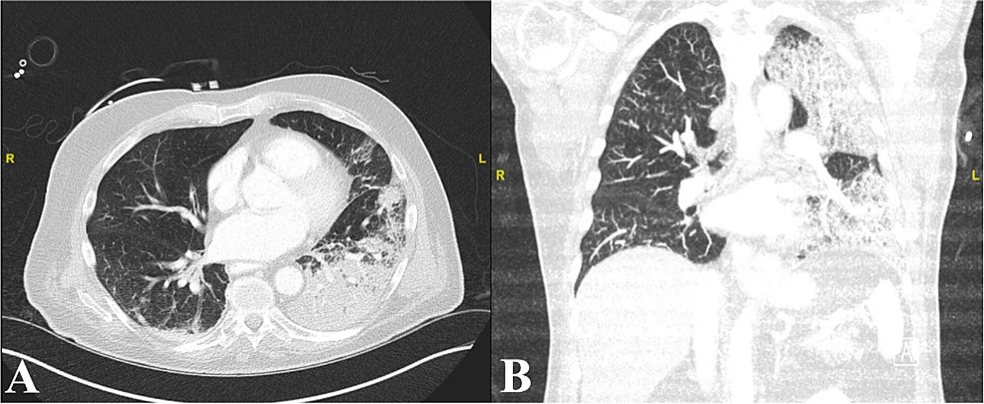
CT of the thorax on axial (A) and coronal (B) planes showing extensive consolidation of the left lung consistent with multifocal pneumonia
